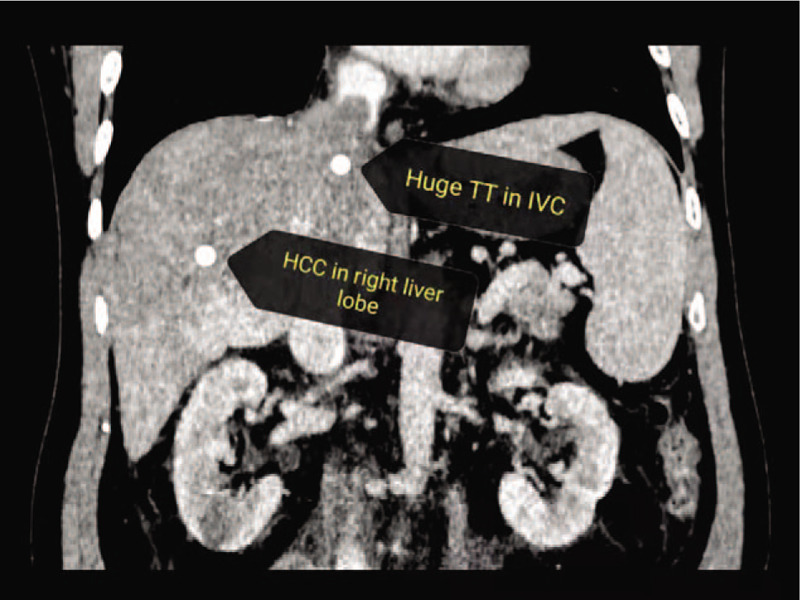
Computed tomography imaging of primary hepatocellular carcinoma and metastatic cardiac mass. HCC = hepatocellular carcinoma, TT = tumor thrombus, IVC = inferior vena cava.
